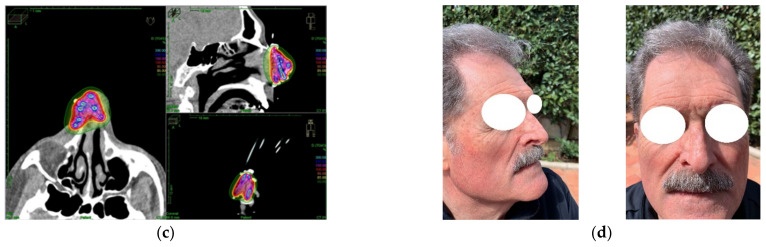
(a) MRI with initial diagnosis. (b) Implant with plastic tubes and buttons sutured to the skin. (c) Treatment plan with isodoses. (d) Cosmetic outcome 1 year after IRT. The arrow indicates the primary lesion of the nose vestibule.
